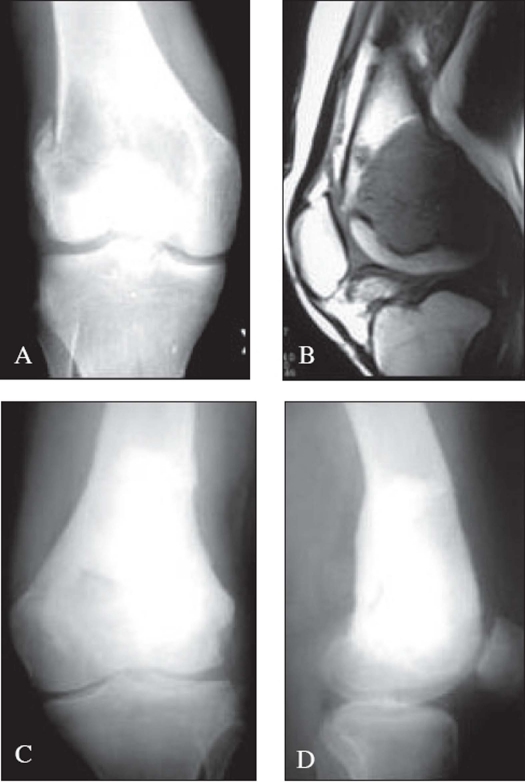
A) X-rays (A.P.) of the knee in a 17-year-old male with GCT of the distal femur with pathological fracture of the lateral femoral condyle. B) MRI of the same patient showing the pathological fracture and articular cartilage free of tumor. C), D). 7 month post operative x-rays (A.P. and lateral) of the same patient treated with curettage, bone graft and bone cement
